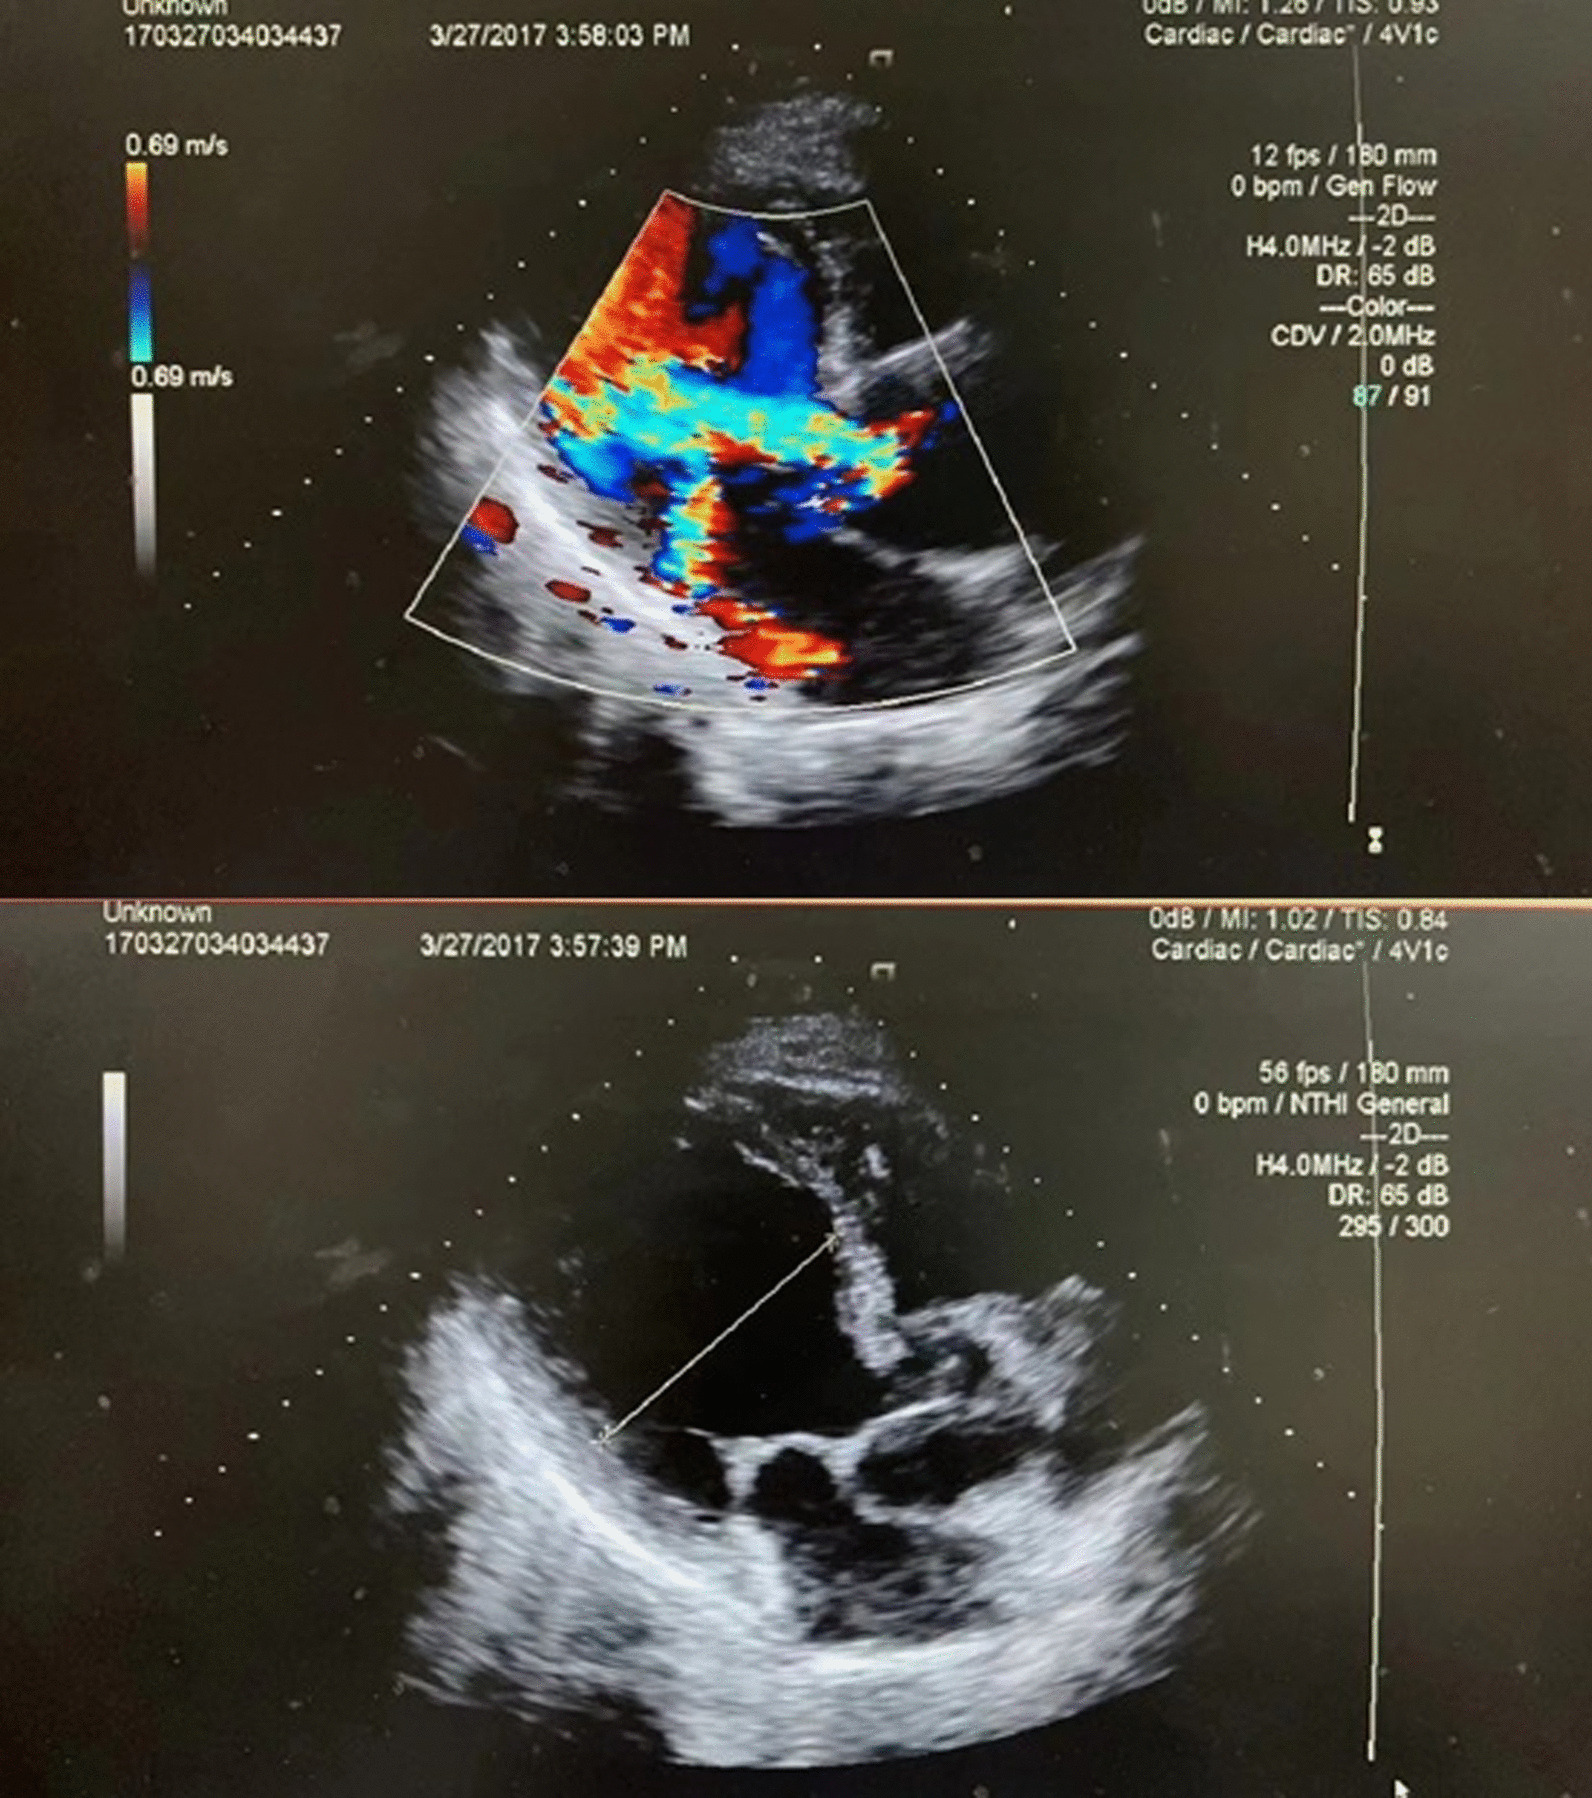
The patient’s echocardiographic results in 2017
